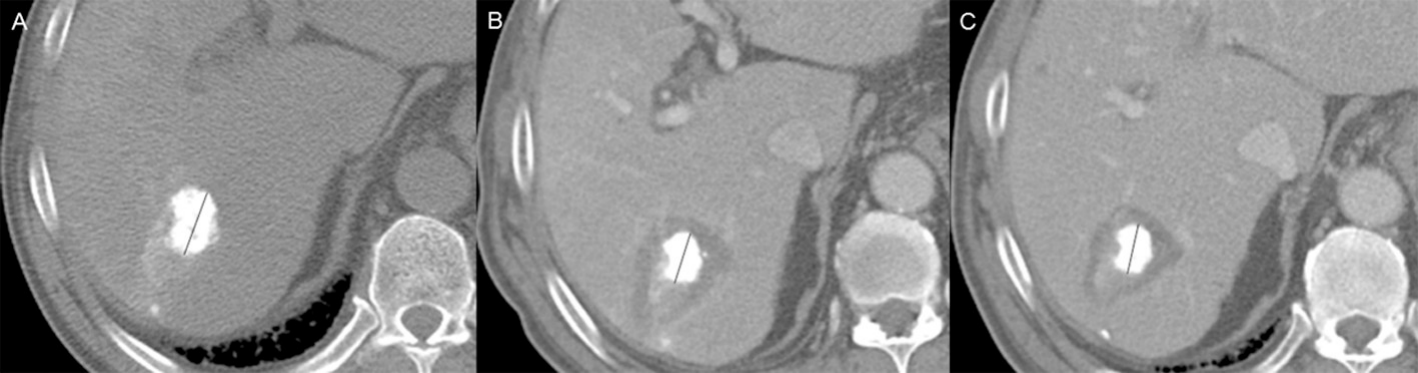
46 year-old man with hepatocellular carcinoma. (a) After transcatheter arterial chemoembolization (TACE), concentrated iodized oil delineates tumor on post-TACE CT (longitudinal tumor diameter, 27.8 mm). (b) Following completion of sequential radiofrequency (RF) ablation, low-attenuation ablation zone encircles index tumor. Tumor size diminished on post-ablation CT (decline in longitudinal tumor diameter: 27.8 mm → 20.7mm; 25.5% post-ablative reduction rate) for post-ablative volume reduction rate of 60.1%. (c) Stability of tumor confirmed by follow-up CT scan 1 month after RF ablation (20.7 mm → 20.4 mm).
